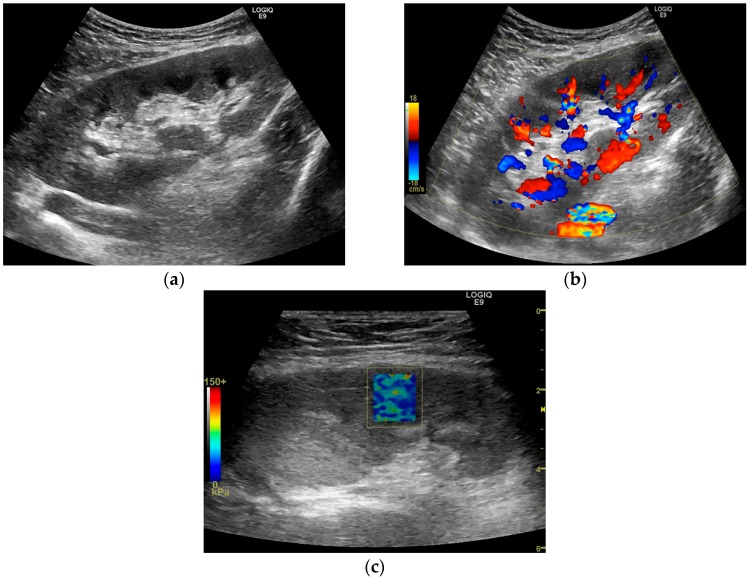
Ultrasound evaluation of a male patient, 27 years old, with normal weight, evaluated at 2005 days after transplant. The kidney allograft function was stable, and the patient was with normal dose of calcineurin inhibitor. The allograft volume was 245 mL, a parenchymal thickness of 12.9 mm, a cortical thickness of 6.3 mm, and normal echogenicity (a). Doppler signal was globally present (b) with all RIs (RI = resistivity index) lower than 0.7 (0.51 at upper interlobar artery, 0.63 at the medium interlobar artery, and 0.56 at the lower interlobar artery). The SWE elastogram box show homogenous colors around low shiftiness indicating the presence of stiff tissue (c) with a median value of YM equal with 37.27 kPa for kidney cortex and 14.31 kPa for kidney medulla and a low variability among measurements on both kidney compartments (QCV equal with 11.13% for cortex and 7.36% for medulla).
